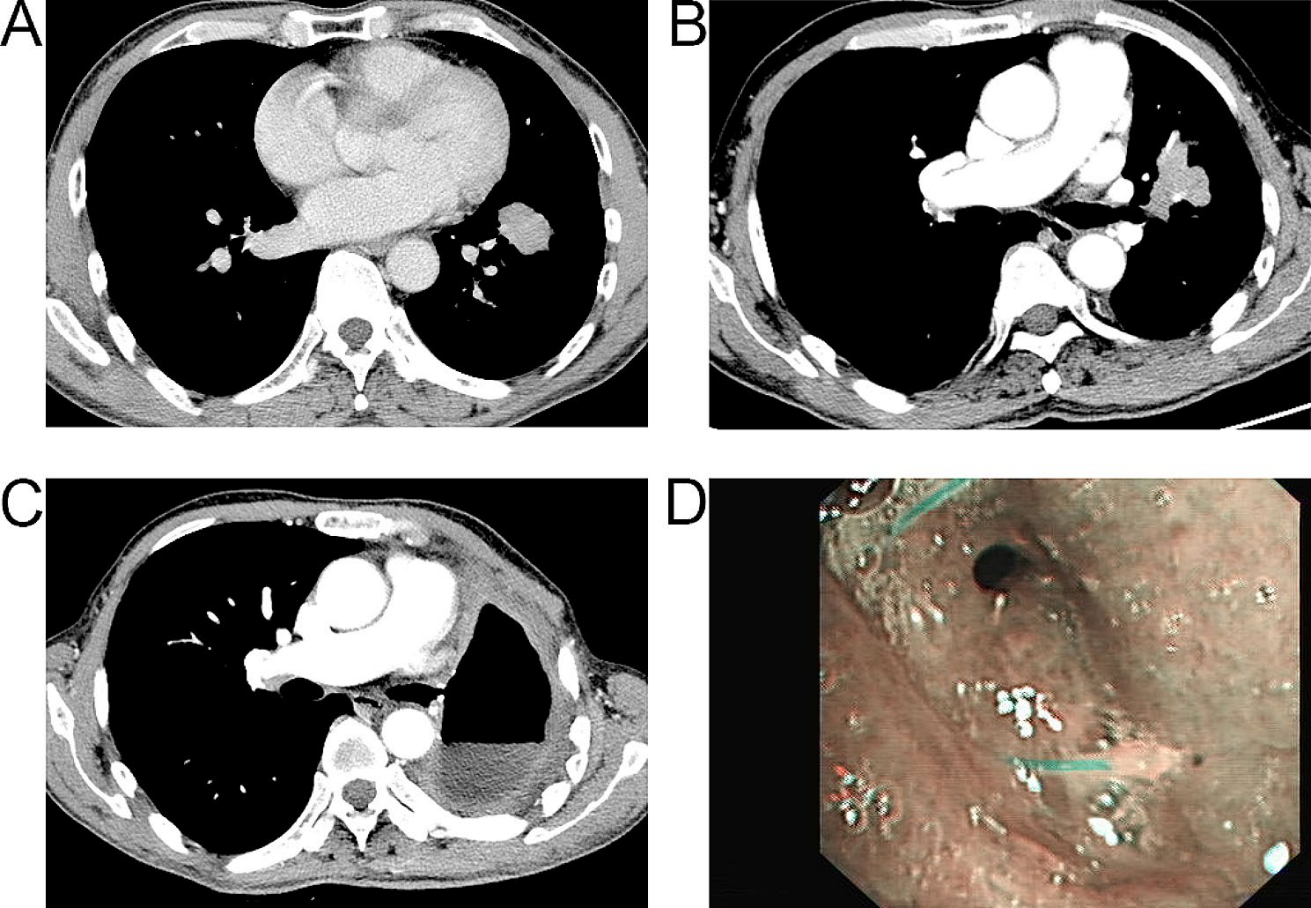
A. He was diagnosed with lung cancer in September 2018 following discovery of 27 mm×25 mm solid nodule in the left lower lung lobe. B. Lung cancer recurrence was later discovered in August 2021 following discovery of a 37 mm×26 mm mass adjacent to the left upper lung hilar. C-D. Chest CT and fibrobronchial examination revealed a small straight fistula at the left bronchial stump communicating with the left residual cavity
